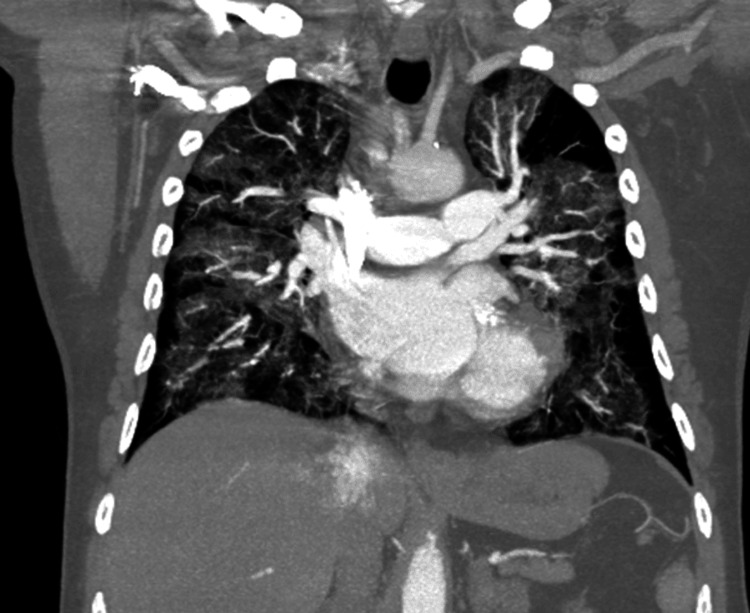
A coronal computed tomography (CT) angiogram of the chest was negative for pulmonary embolism; however, it revealed diffuse bilateral interstitial and airspace opacities
